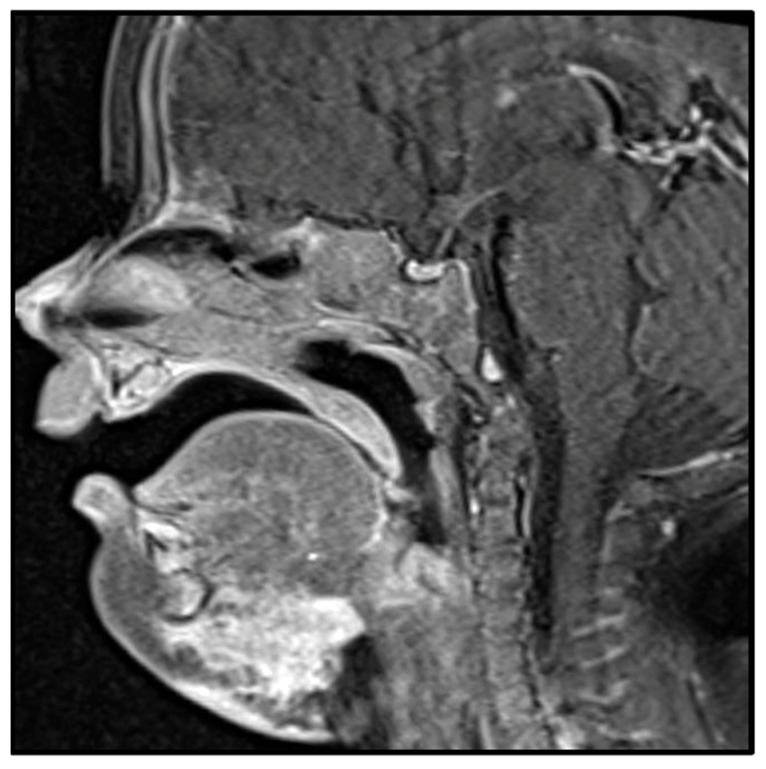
MRI image of kaposiform hemangioendothelioma involving the submental region with subcutaneous and soft tissue involvement.
